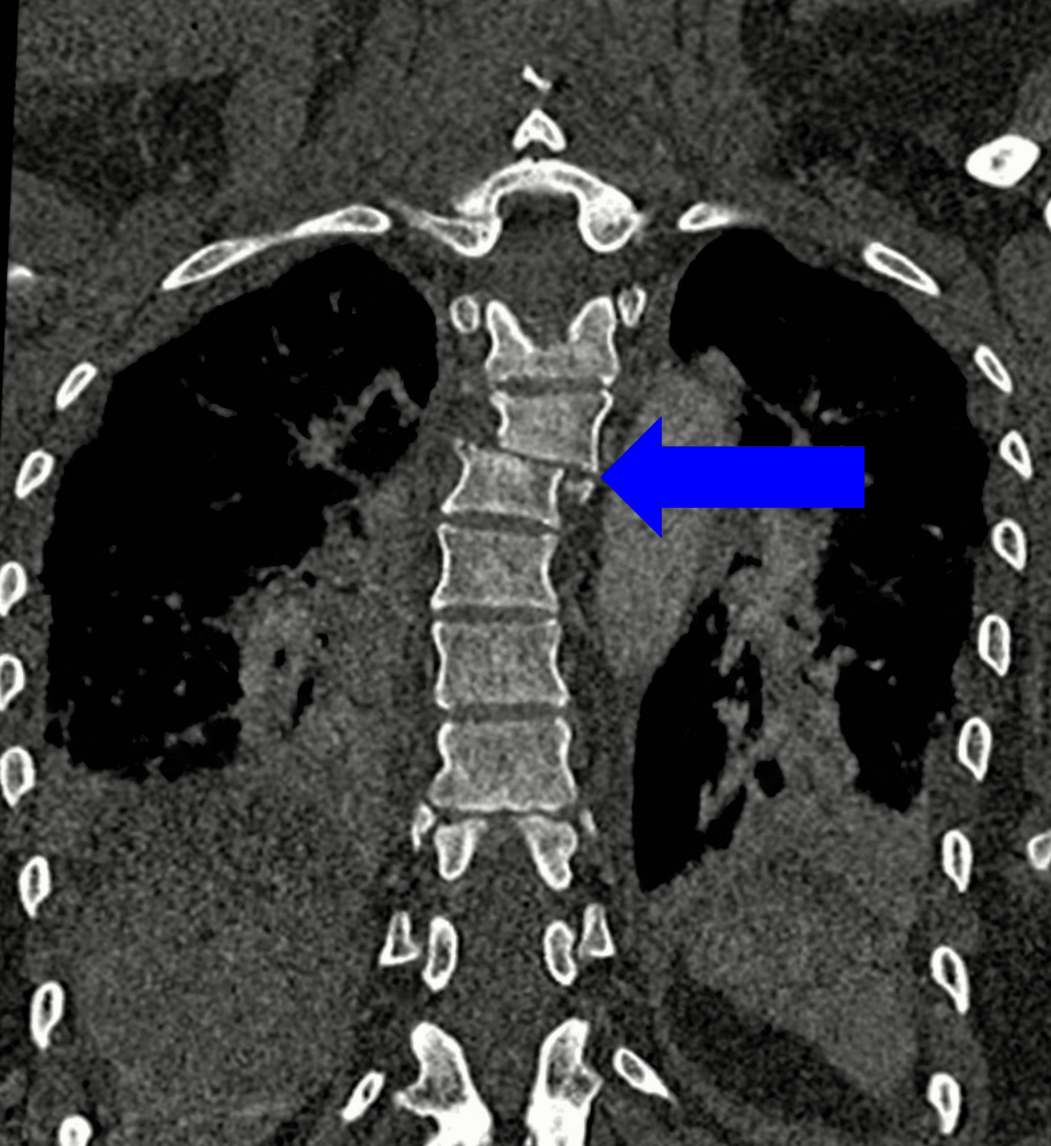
Coronal CT scan of the thoracic spine Coronal CT scan demonstrating the fracture-dislocation of the thoracic spine with significant lateral translation of the vertebral column.
